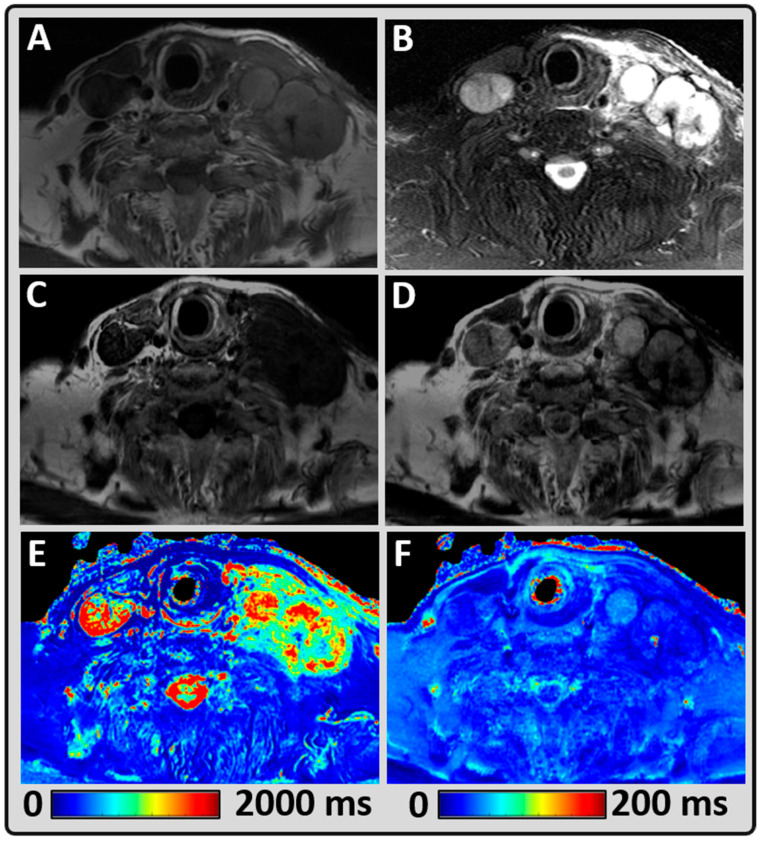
Representative MRI data from a 76-year-old male patient post-thyroidectomy with a bilateral metastatic supraclavicular lymph node. (A,B) Conventional qualitative T1- and T2-weighted (w) images depicting the metastatic tumors; (C,D) Synthetically generated T1w and T2w images using MAGiC; (E,F) MAGiC-generated quantitative T1 and T2 maps.
